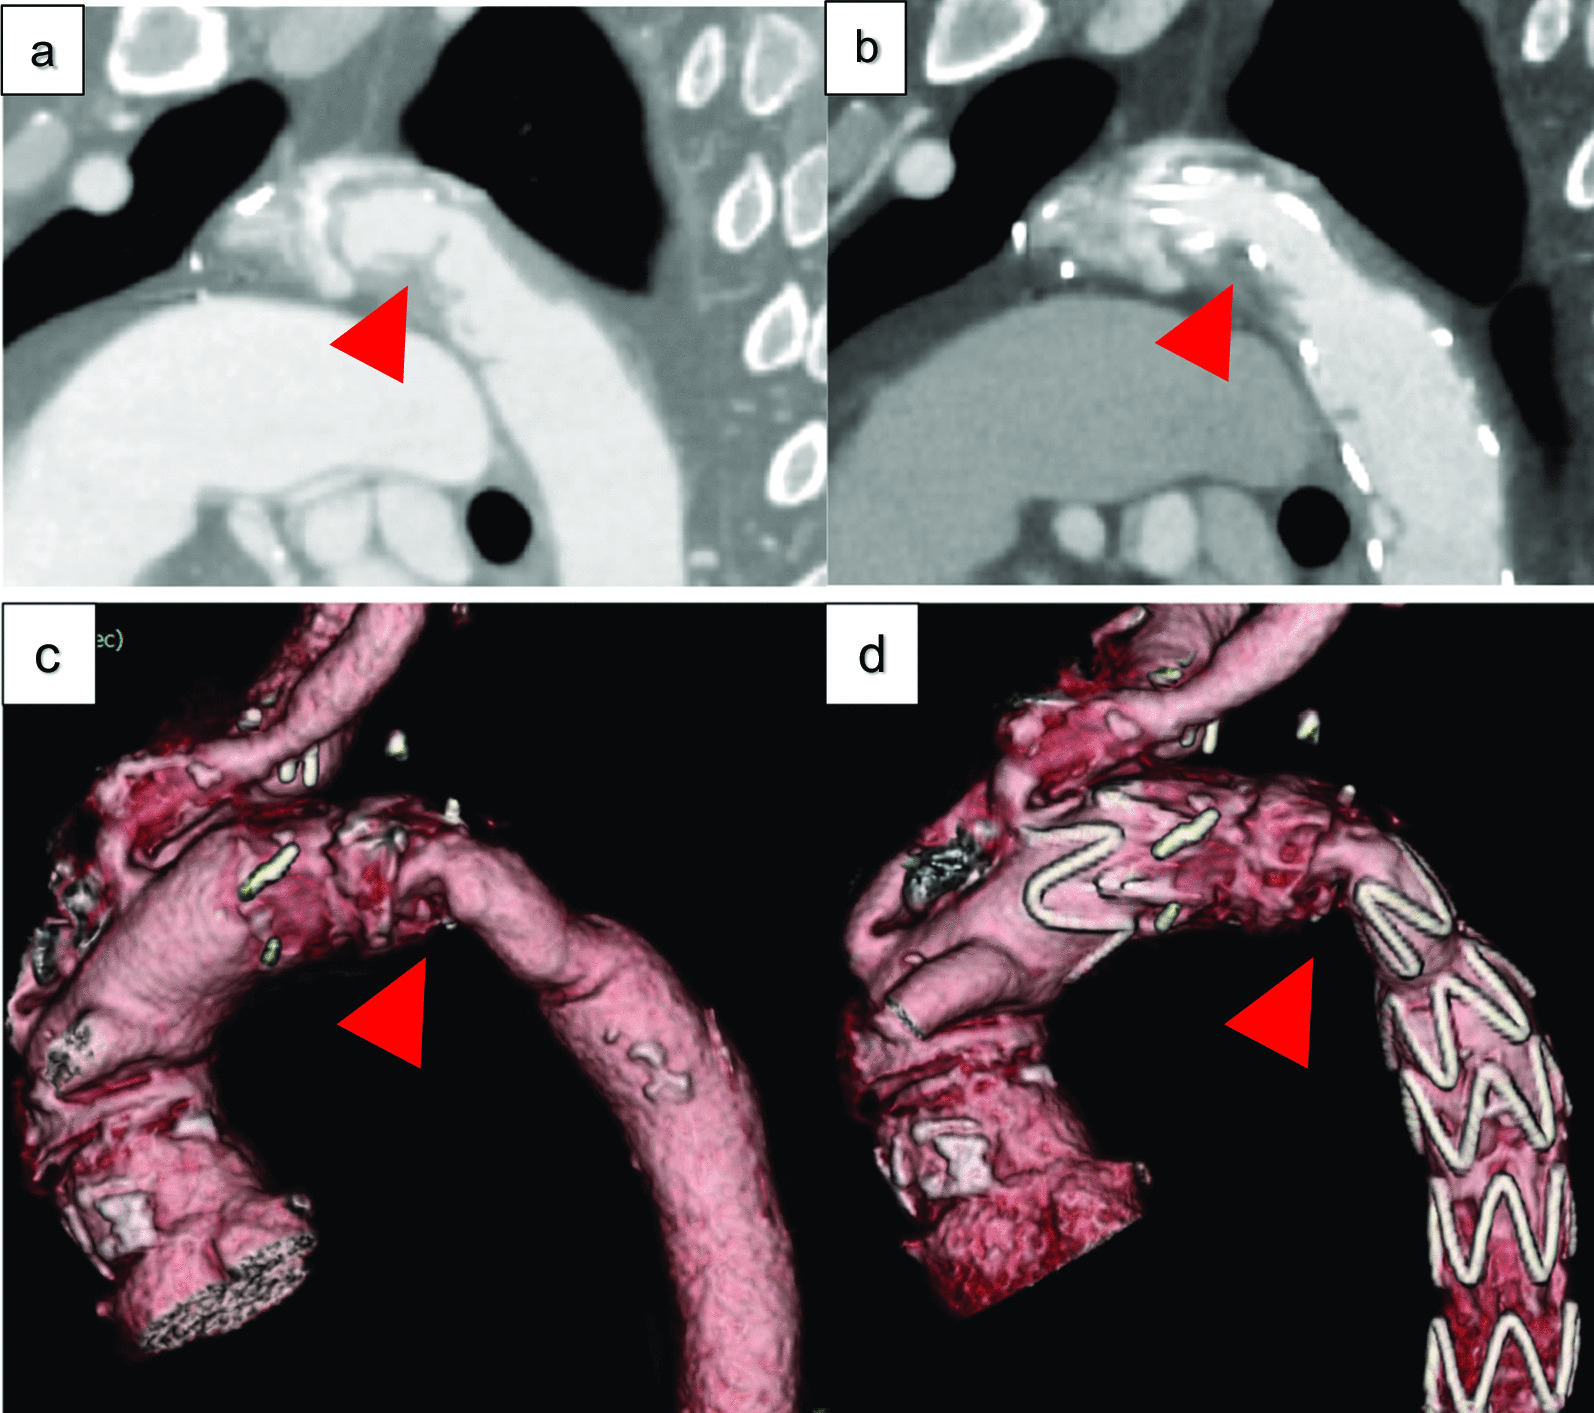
Preoperative and postoperative computed tomography. Narrowing artery within Elephant Trunk (Red arrows). a, c Before TEVAR, b, d After TEVAR
